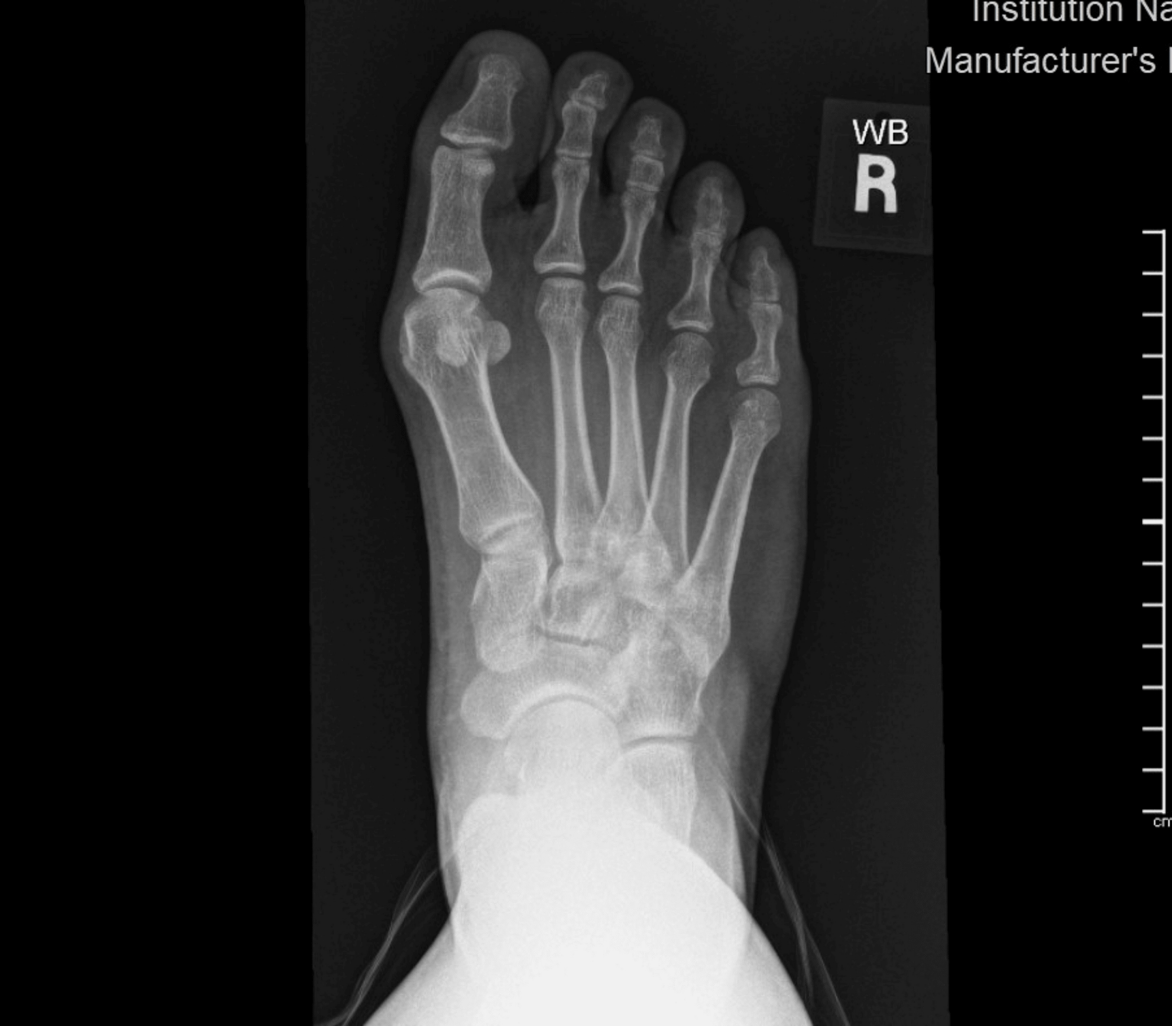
Pre-operative weightbearing AP radiograph AP: anterior-posterior
